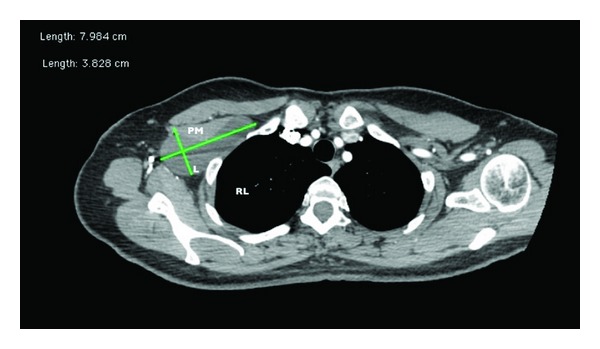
Chest CT with intravascular contrast, axial view. PM: pectoral muscle; L: lymphocele; RL: right lung.
